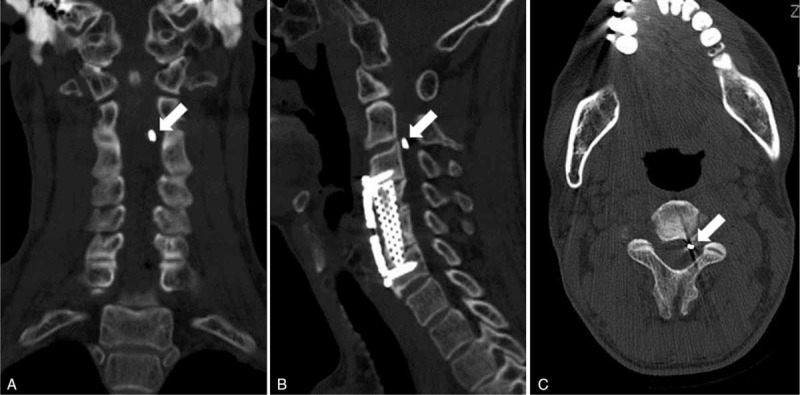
In 2-year follow-up, CT revealed that the metal fragment remained in C2/3 canal and was close to left foramen, without apparent neurological compression. CT = computerized tomography.
